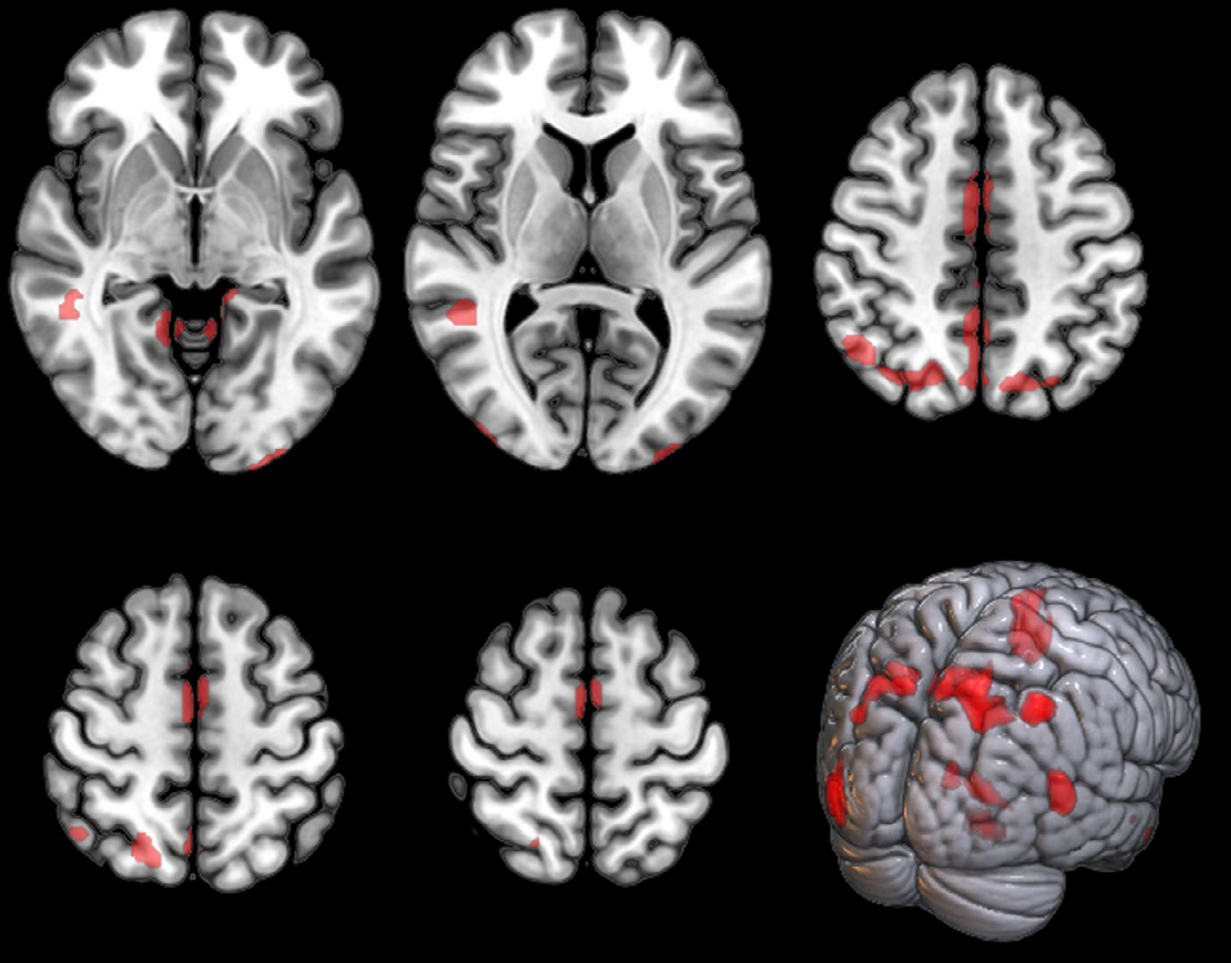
Clusters of relative hypometabolism of male as compared to female ALS patients are marked in red and are reported on axial sections of a brain magnetic resonance imaging template and on the brain surface of a glass brain rendering (bottom right).
